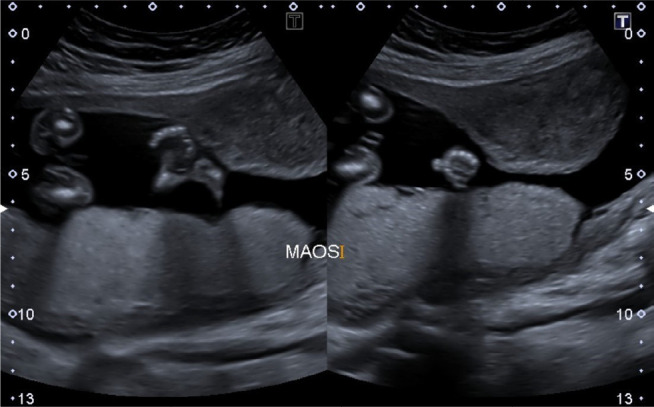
Obstetric ultrasound demonstrating bilateral polydactyly.
